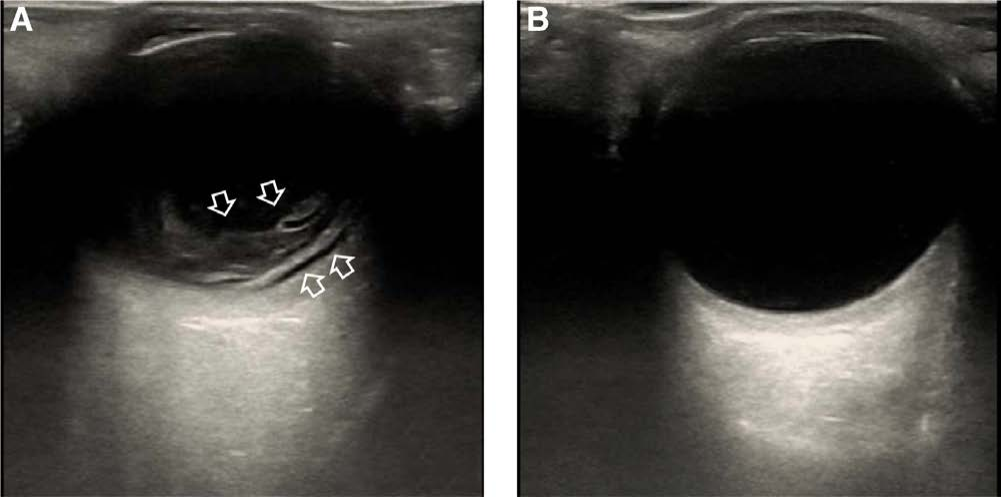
(A) Bedside ultrasonography showed numerous acoustic shadows in the vitreous cavity and retinal detachment of the right eye; (B) the left eye was normal.
